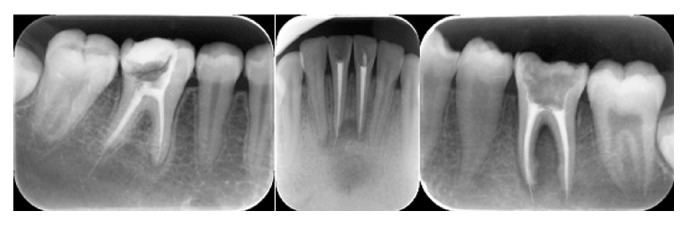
Extrusion of gutta-percha beyond the radiographic apex.
